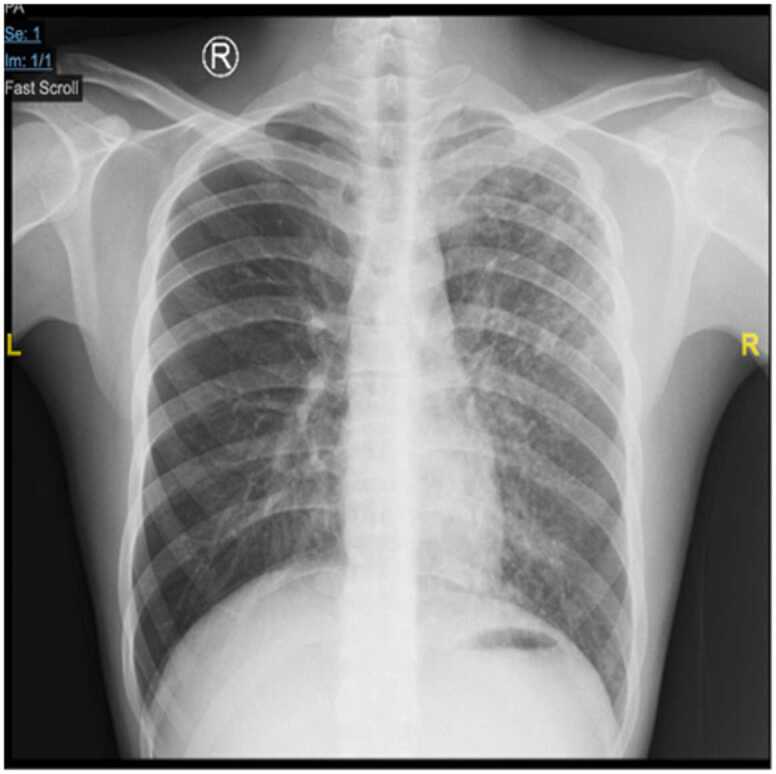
Chest X-ray on admission. Infiltrates were seen in the field above to below the left lung.
